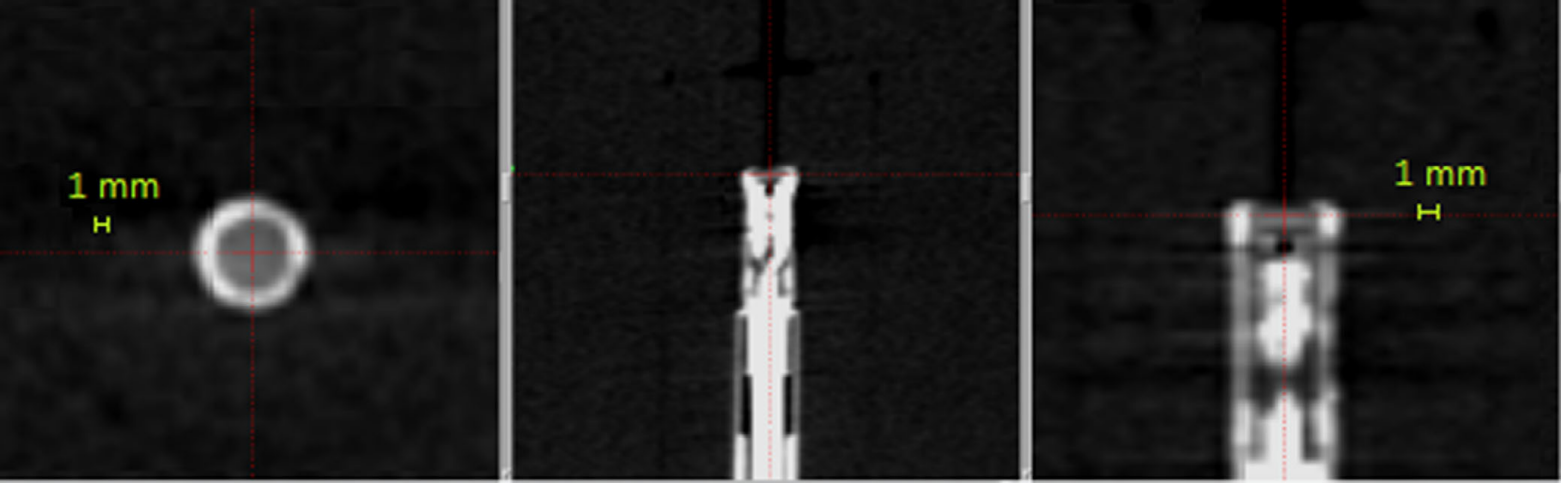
Cone beam computed tomographyCBCT of the MicroDiamond detector and centering it at the unit center point.
